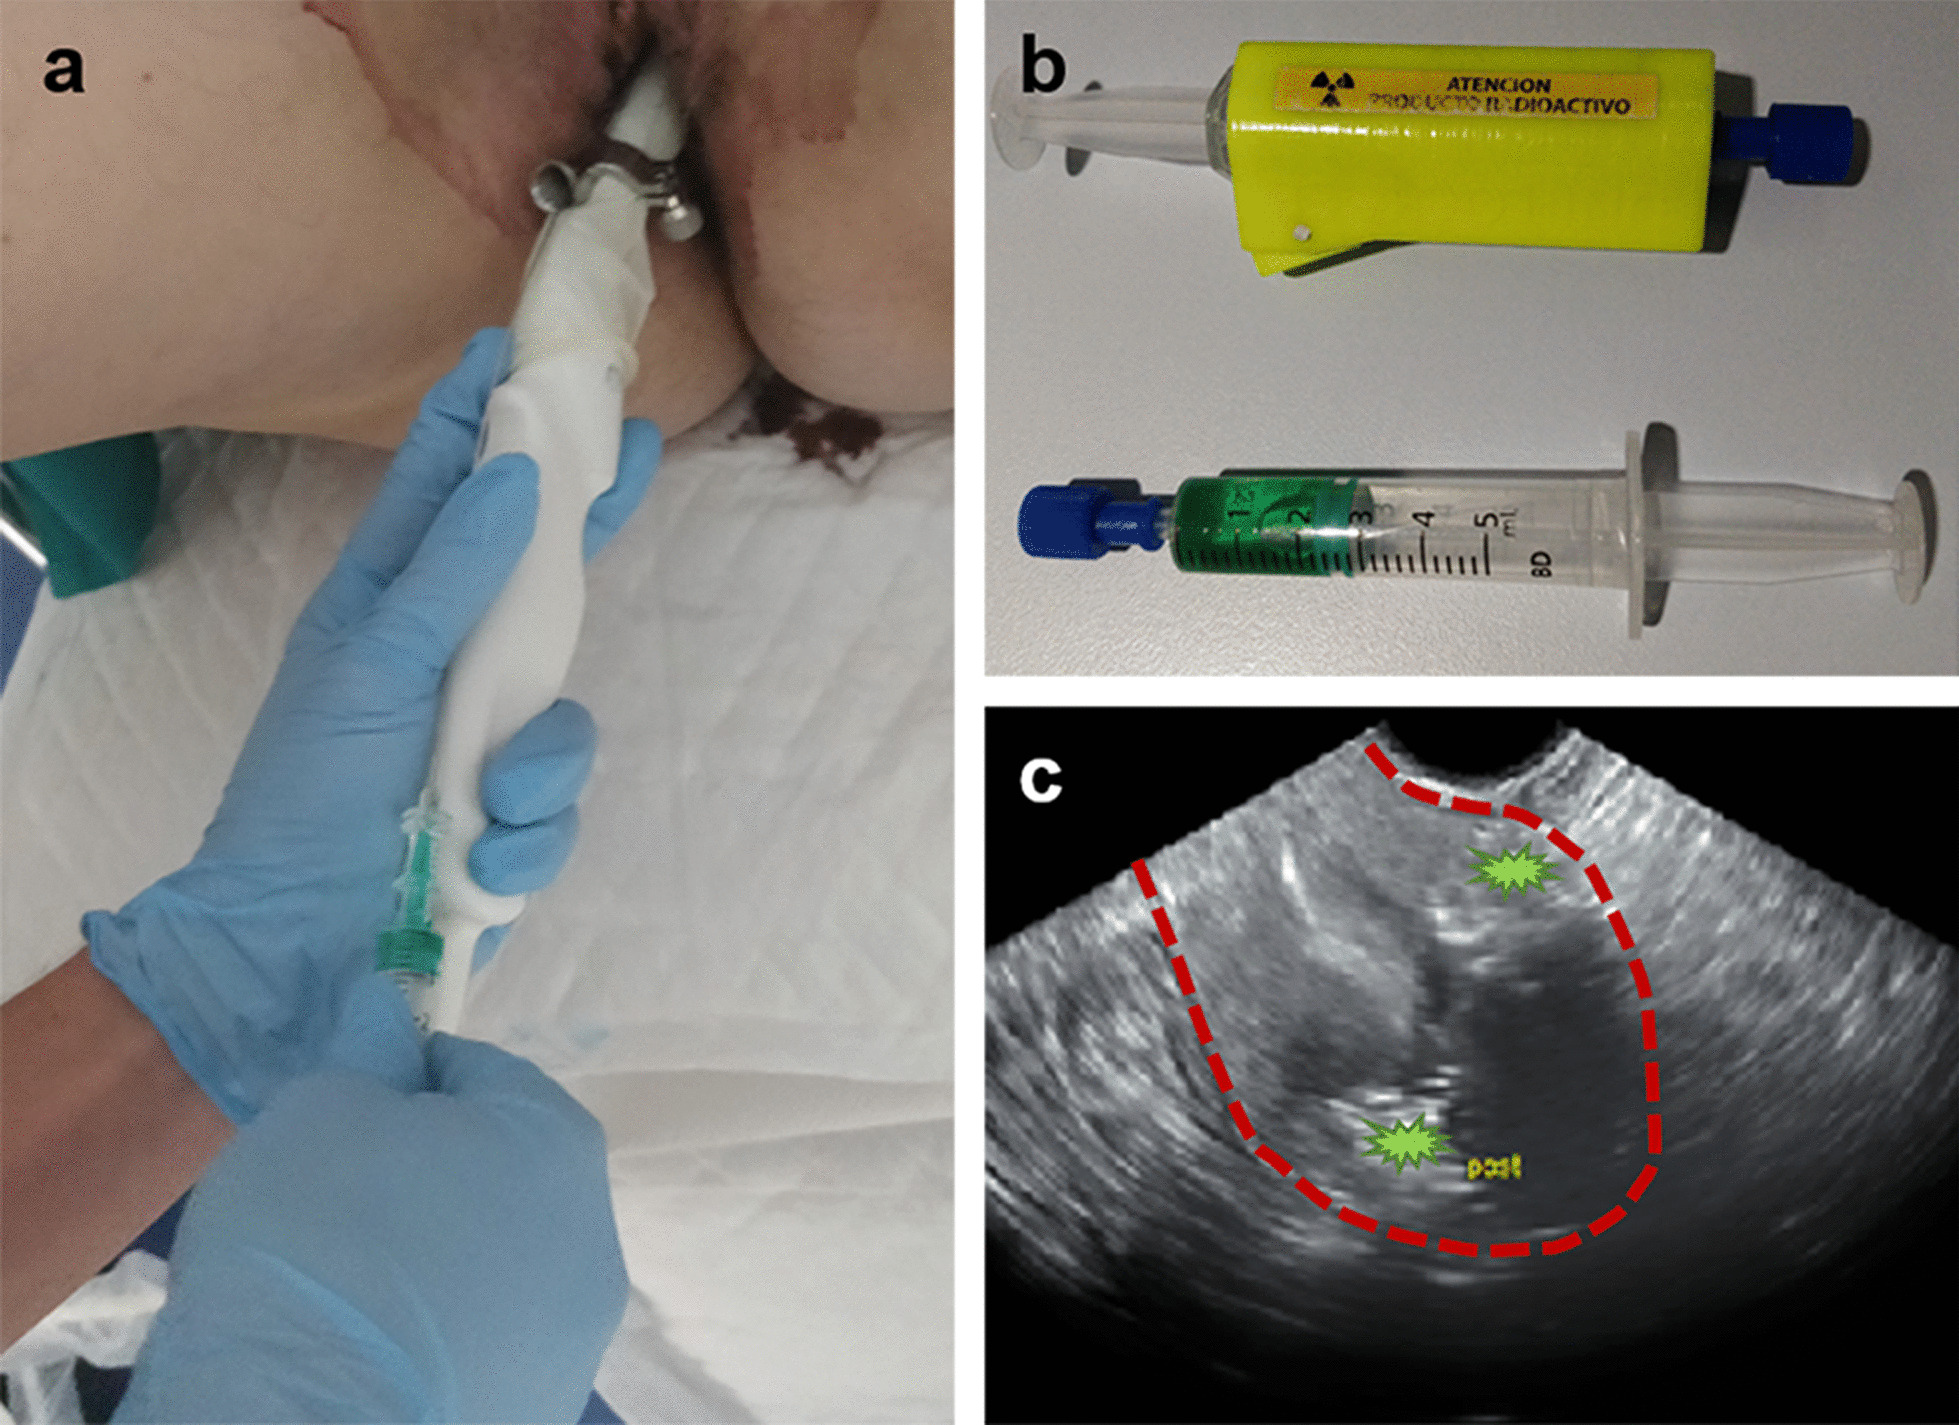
Transvaginal ultrasound myometrial injection of the hybrid tracer (a). Hybrid tracer (ICG-[^99m^Tc]Tc-nanocolloid) prepared for the injection (b). Tracer accumulation (green asterisk) in the anterior and posterior walls of the myometrium. Intermittent red line delimitates the uterus. Red double-headed arrow indicates the endometrium (c)
